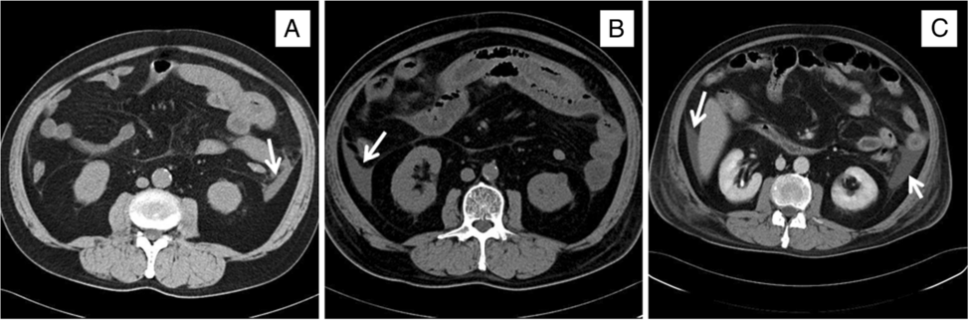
Computed tomography of the abdomen. (A) Computed tomography of the abdomen performed on admission, showing diffuse thickening of the bowel wall with target signs in the small intestine and ascites (arrow). (B) Computed tomography of the abdomen performed on day 9, showing severe edematous and dilatational changes in the small intestine and ascites (arrow) before treatment with steroids and cyclophosphamide. (C) Computed tomography of the abdomen performed on day 29 before treatment, showing ascites (arrows) that remained after treatment with steroids and cyclophosphamide. However, the severe edematous changes in the small intestine were slightly improved relative to day 9.
